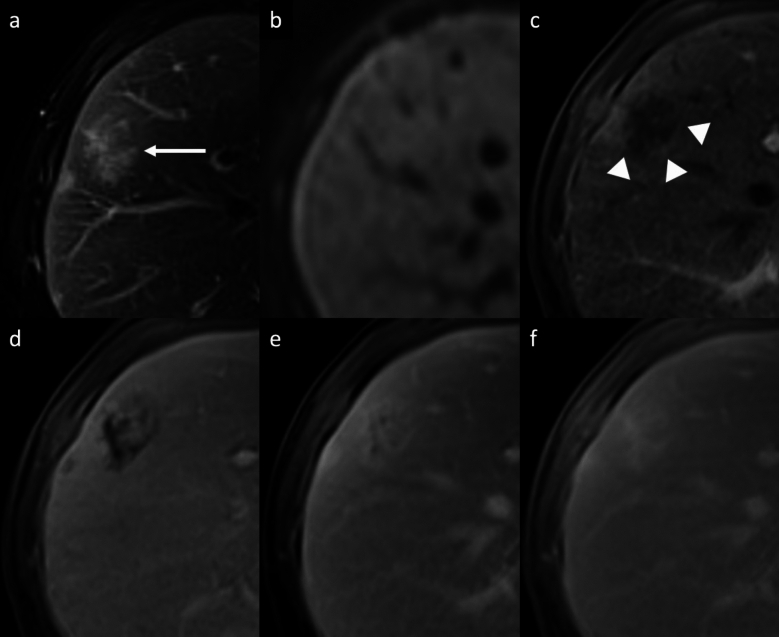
Hepatic peliosis in a 35-year-old woman with sinusoidal obstruction *syndrome. *a Axial fat-suppressed T2-weighted MRI shows a subcapsular focal liver lesion in the right lobe (arrow) with heterogeneous high signal intensity, and with an isointense signal on diffusion-weighted imaging (b). Extracellular contrast agent-enhanced MRI demonstrates a mild peripheral discontinuous enhancement on arterial phase (arrowheads, c), followed by a progressive and centrifugal enhancement on portal venous (d) and delayed phases at 3′ (e) and 5′ (f), showing a central focus of contrast pooling. Biopsy of the lesion confirmed the diagnosis of hepatic peliosis
